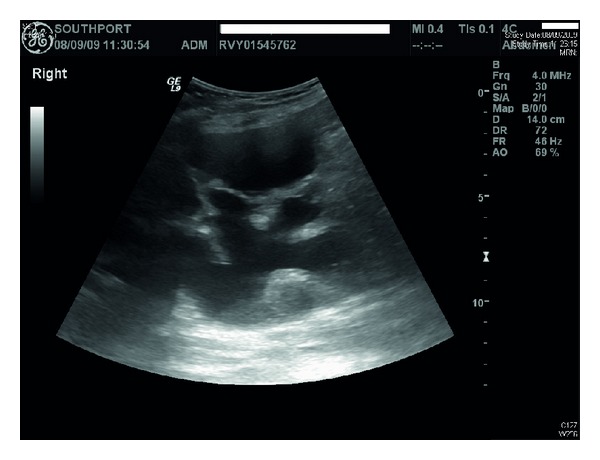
Ultrasound of urinary tract performed in 2009 revealed marked hydronephrosis of right kidney and bilateral renal cortical cysts.
